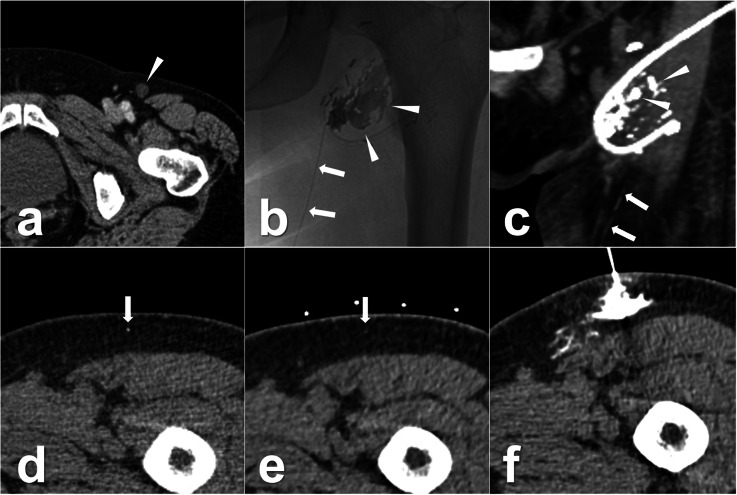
Images from a patient who underwent a melanoma resection on the left leg 4 months before. a A suspicious relapse of one left inguinal lymph node (white arrowhead). Then, a left inguinal lymphadenectomy was performed, and the pathological result confirmed the lymph node metastasis. After this surgery, the continuous LL was observed with an average output of 100 mL/day. Owing to the ineffective surgical revision, the TL was performed and definite iodinated oil extravasation (white arrowheads) from one lymphatic vessel (white arrows) was found using both fluoroscopy (b) and a sequential CT scan, obtaining coronal (c) and axial (d) reconstructions. Ten days after TL, percutaneous ALVS was performed due to there being no regression of the drainage. e In the preoperative CT scan, the afferent lymphatic vessel (white arrowhead) could still be identified. f Afterwards, a successful puncture was achieved, and 1 mL of contrast fluid was injected that showed an ideal distribution around the target lymphatic vessel. Then, 1.0 mL 95% ethanol was injected. One day later, the leakage ceased completely, and the drainage was removed. The patient was discharged on the same day. *ALVS* Afferent lymphatic vessel sclerotherapy, *CT* Computed tomography, *LL* Lymphatic leakage; *TL* Transpedal lymphangiography
